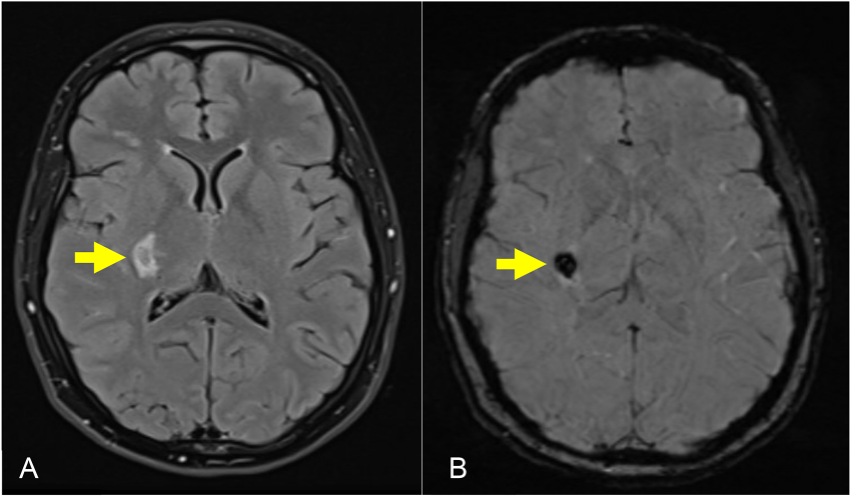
Magnetic resonance imaging (MRI) brain with (a) axial T2 fluid-attenuated inversion recovery (FLAIR) and (b) axial susceptibility-weighted imaging (SWI) sequences demonstrating an intracerebral hemorrhage (ICH) in the right posterior lentiform nucleus, posterior limb of the internal capsule, and lateral thalamus.
